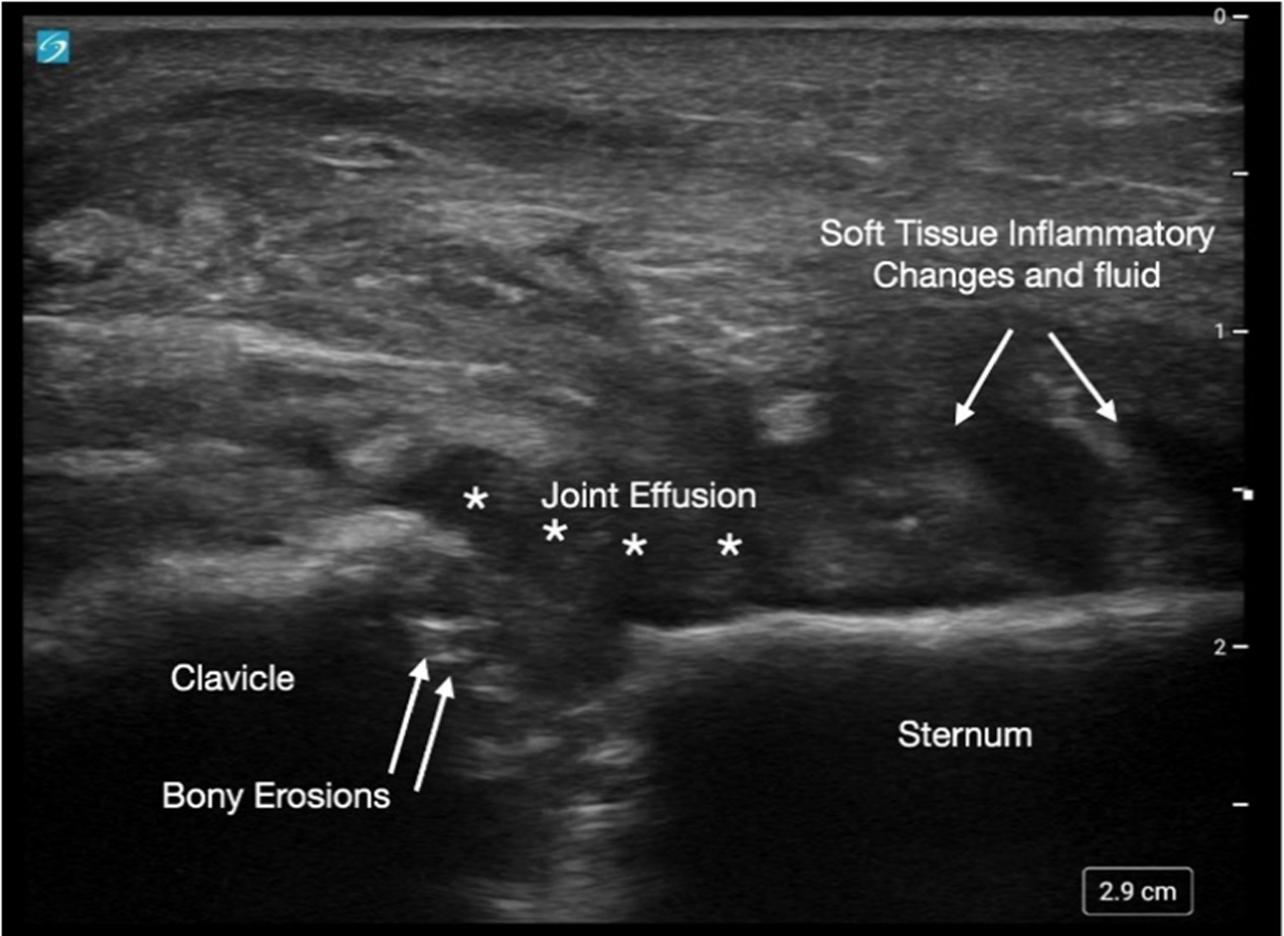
Point-of-care ultrasound of the sternoclavicular joint revealing intra-articular effusion and capsular distention.
